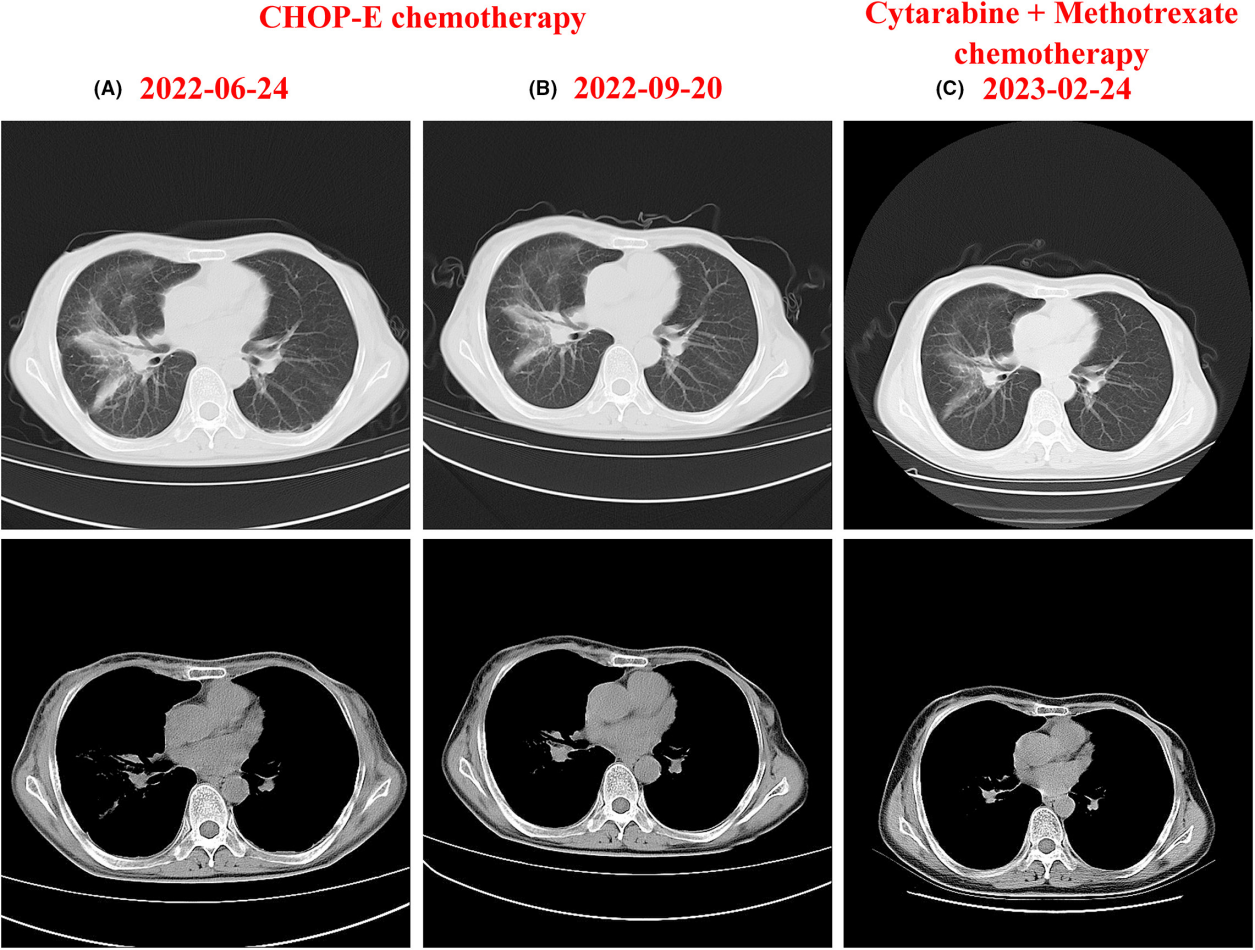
Chest CT scans following CHOP‐E and cytarabine + methotrexate chemotherapy regimens. (A) CT scan after one cycle of CHOP‐E chemotherapy. (B) CT scan after four cycles of CHOP‐E chemotherapy. (C) CT scan after two cycles of cytarabine (150 mg, Days 1–5) and methotrexate (1500 mg, Day 1) chemotherapy.
